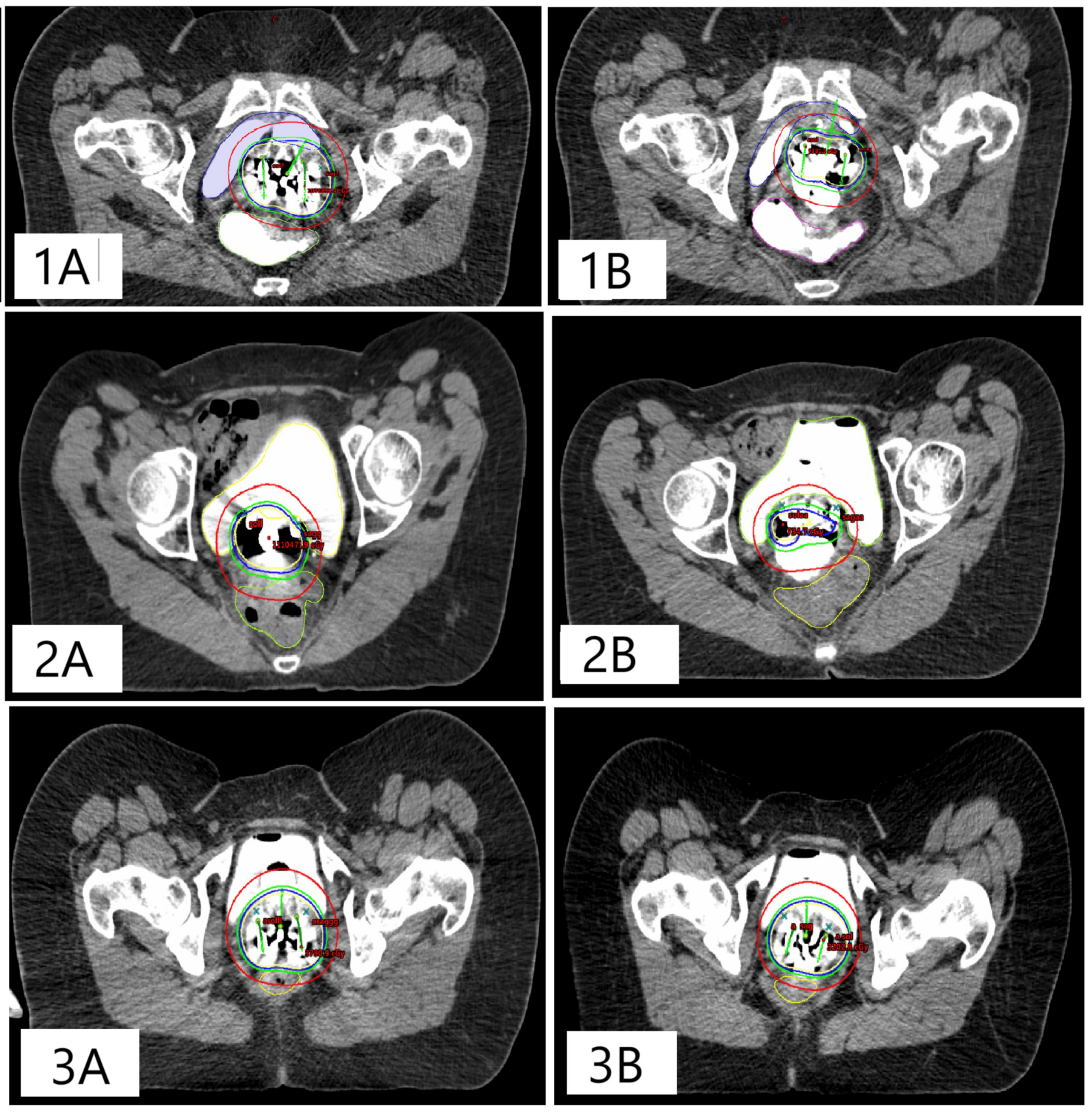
Axial CT images of SP and VGP plan for the three patients The images labeled with “A” next to the patient numbers correspond to the SP, while those labeled with “B” correspond to the VGP plan. Dose distributions: the yellow line represents 100% isodose; the blue line represents 90% isodose; the green line represents 80% isodose; and the red line represents 50% isodose. SP: Standard plan; VGP: Vaginal gauze packing
